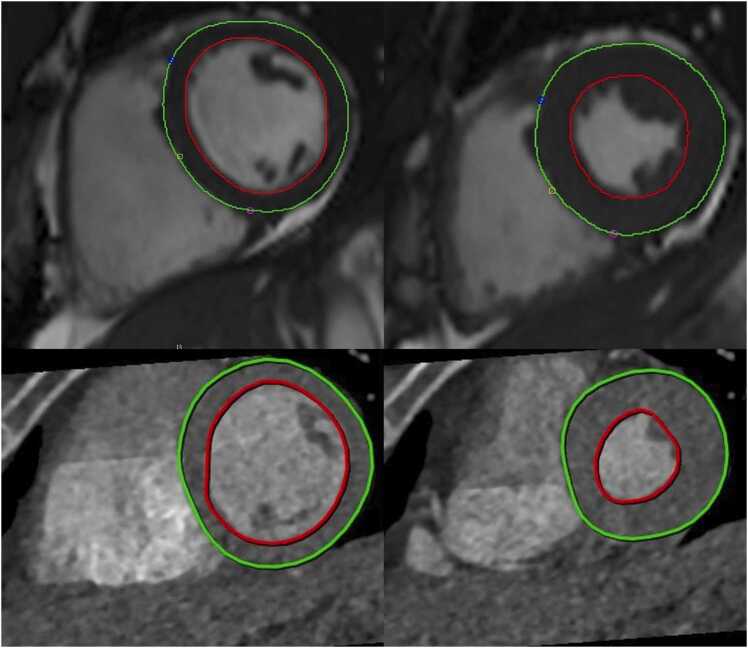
Automated LV segmentations of MRI (top) and CT - standard mode (bottom) in the same patient. ED images are on the left, and ES images are on the right. In this particular case, LVEF was 70 % for MRI and 75 % for CT.
